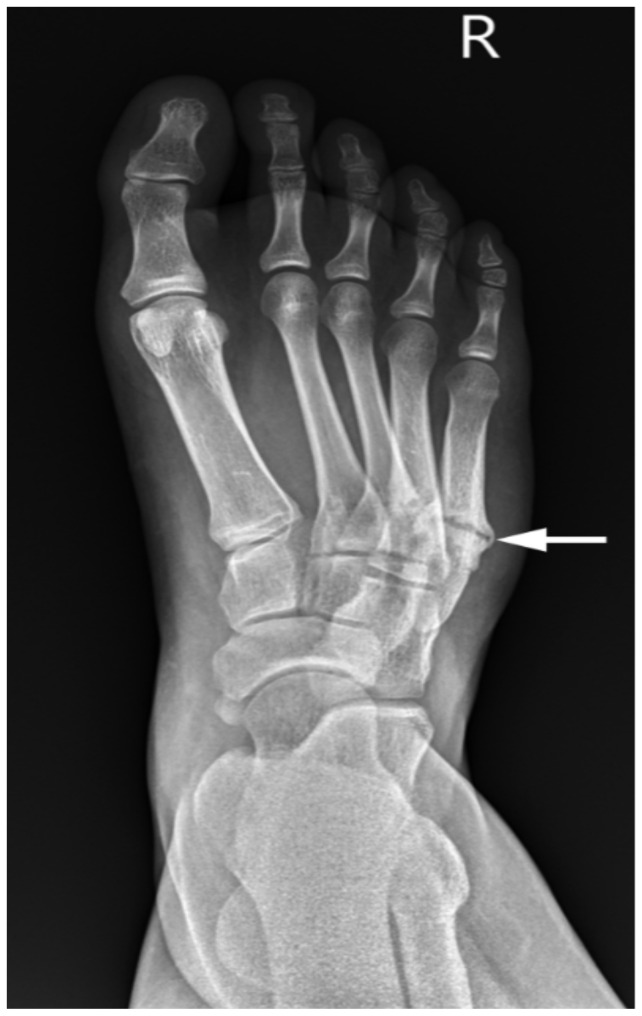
Radiographic features of Stage 4 MSP in the foot. Severe varus deformity of the foot in a 14-year old boy with Type IV hemiplegia, GMFCS II. There are healing fractures of the 4th and 5th metatarsals, from severe chronic overloading. Management to date has been injections of BoNT-A to the gastrocsoleus and tibialis posterior.
